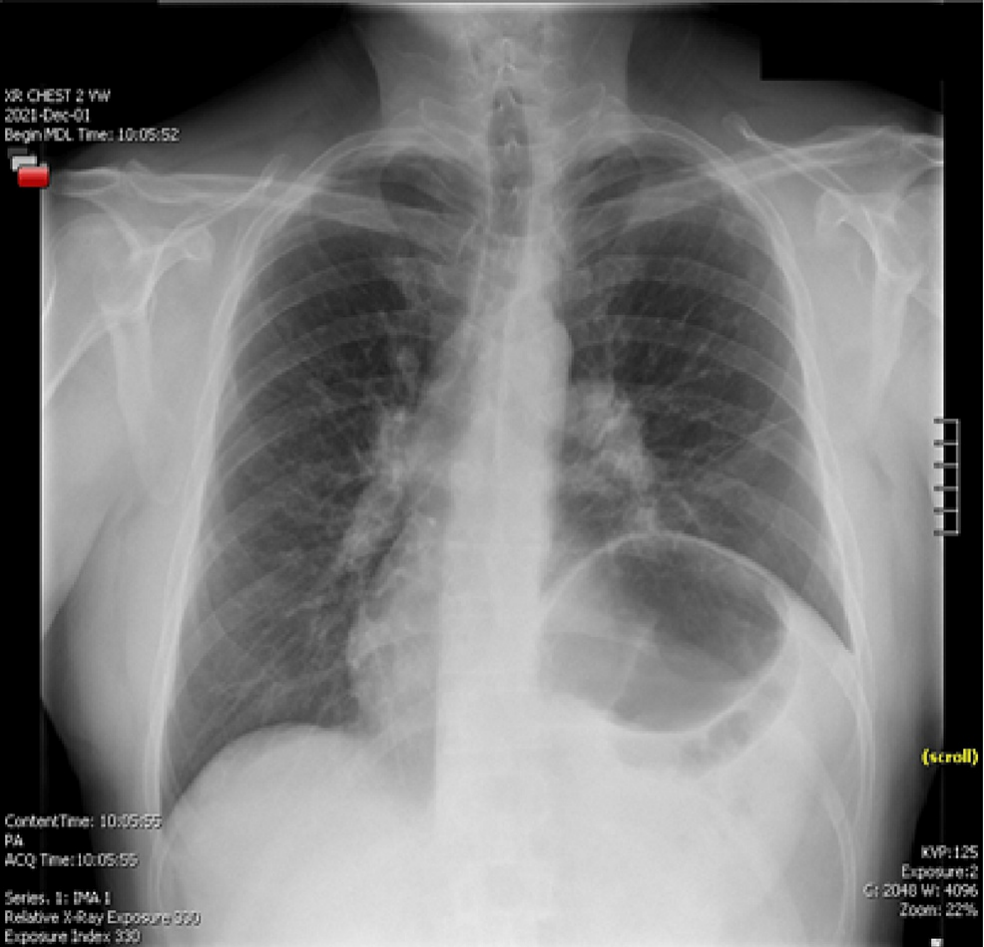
Chest X-ray showing no improvement in left hemidiaphragmatic paralysis after 64 days of initial treatment
